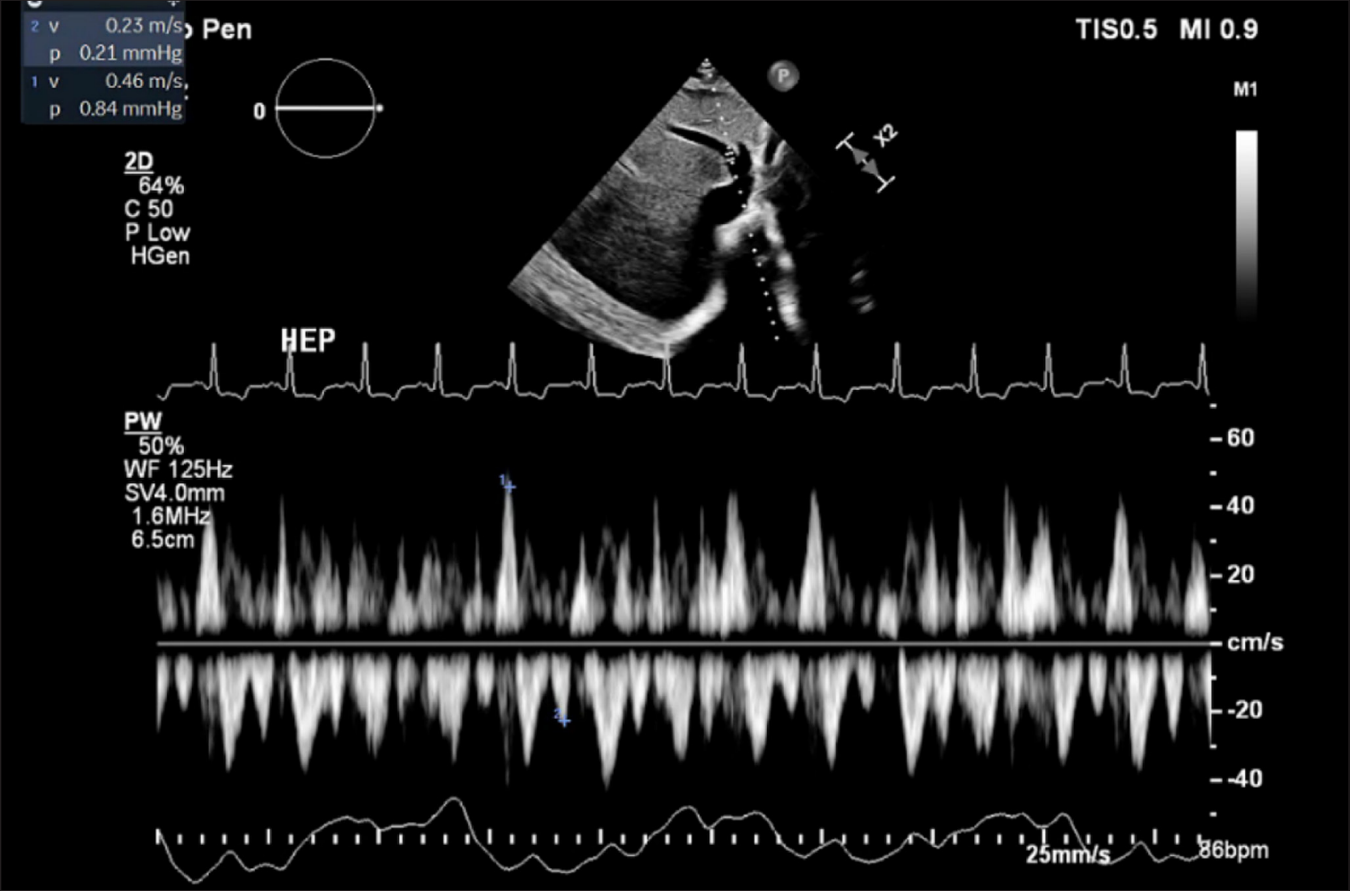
Echocardiogram showing diastolic hepatic vein flow reversal.
